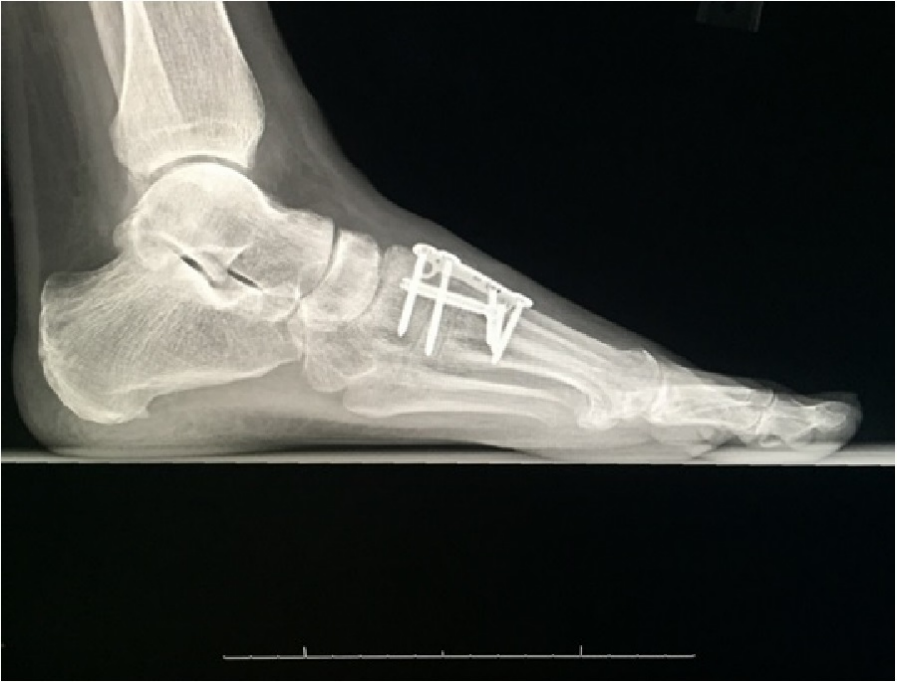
Postoperative weightbearing lateral radiograph of the left foot demonstrating joint-sparing stabilization of the first metatarsal cuneiform joint and Lisfranc joint fixation with a screw
